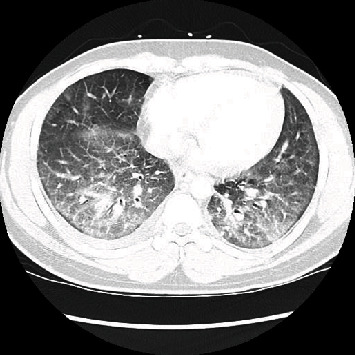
Chest CT: bilateral mixed interstitial and ground-glass infiltrates. Lower lobe predominance. Small right pleural effusion.
